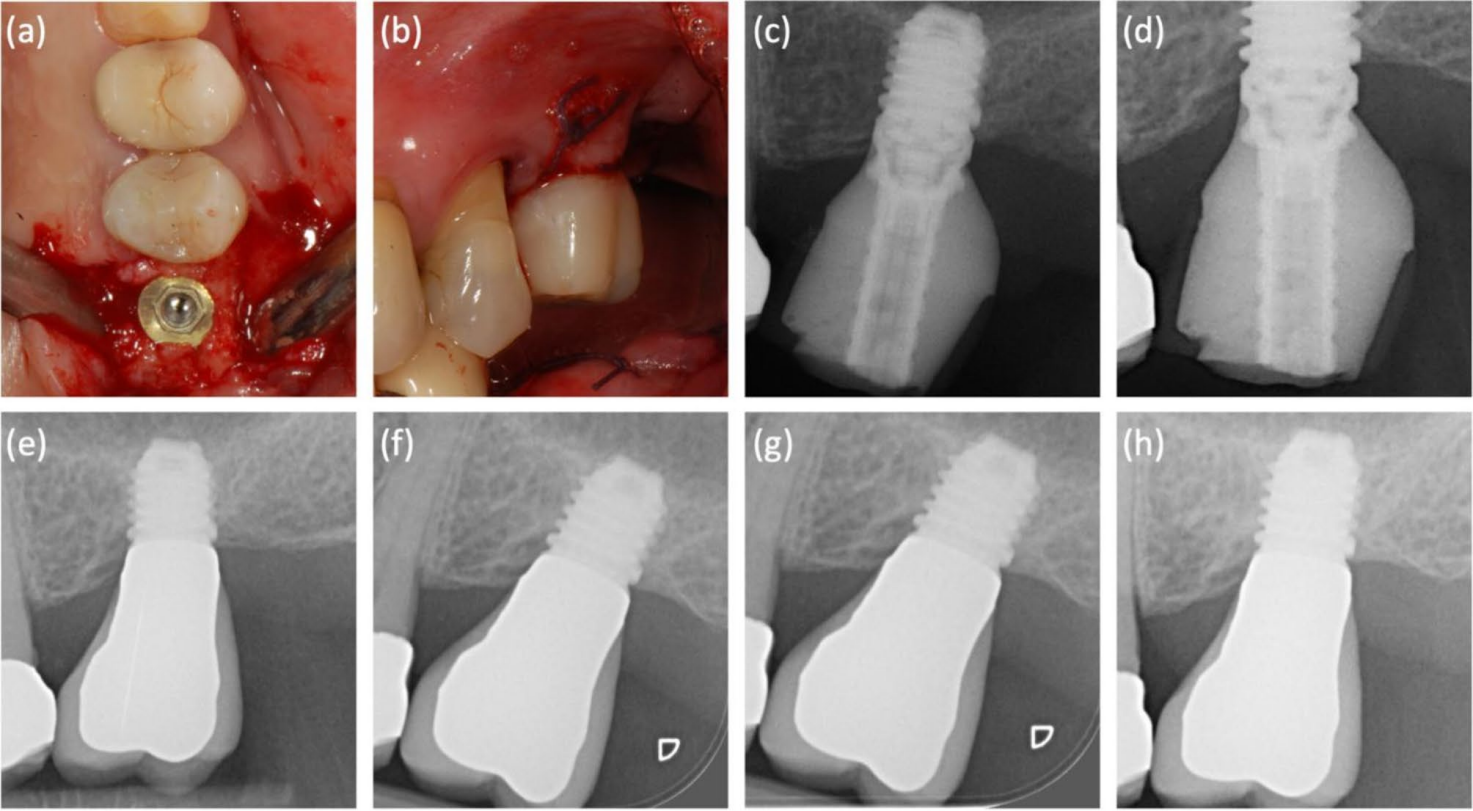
Representative case of extra-short implant (5 mm diameter and height) placed on 2.6 site with immediate non-functional loading. (a) Implant positioning, (b) immediately provisional crown, (c) x-ray at loading, (d) x-ray at 1 year, (e) x-ray at 2 years, (f) x-ray at 3 years, (g) x-ray at 4 years, (h) x-ray at 5 years
